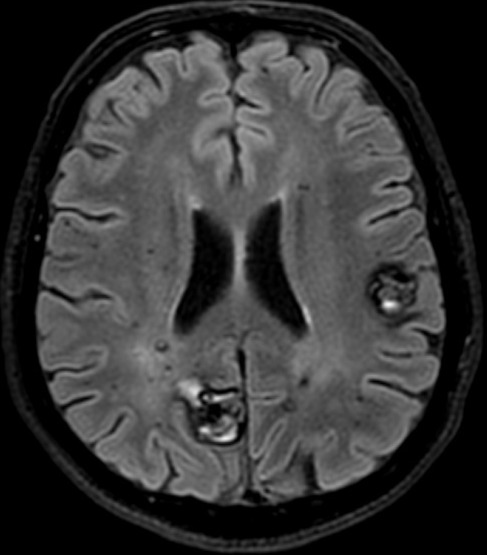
IRM cérébrale, coupe axiale à l’étage supra tentoriel, séquence T2 FLAIR montrant des cavernomes multiples en hyposignal hétérogène « en poivre et sel »
